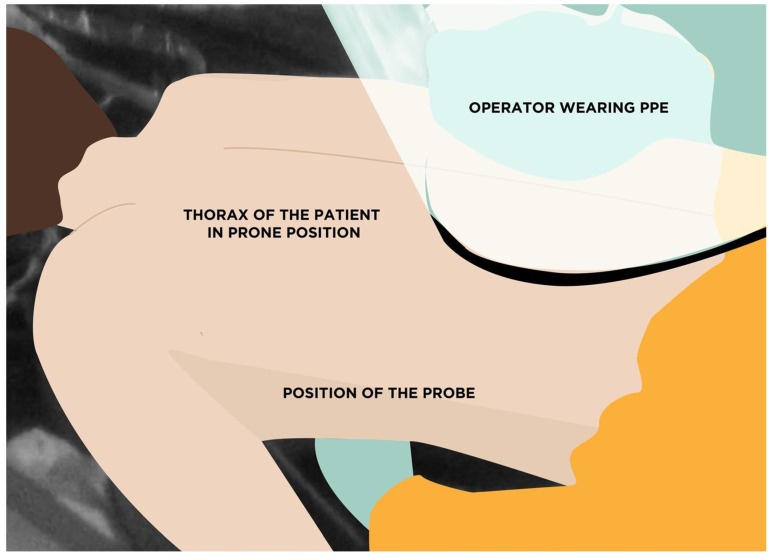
Relative positions of the operator performing echocardiography and the patient in prone position. Abbreviations: PPE, personal protective equipment. Reprinted with permission from [8].
